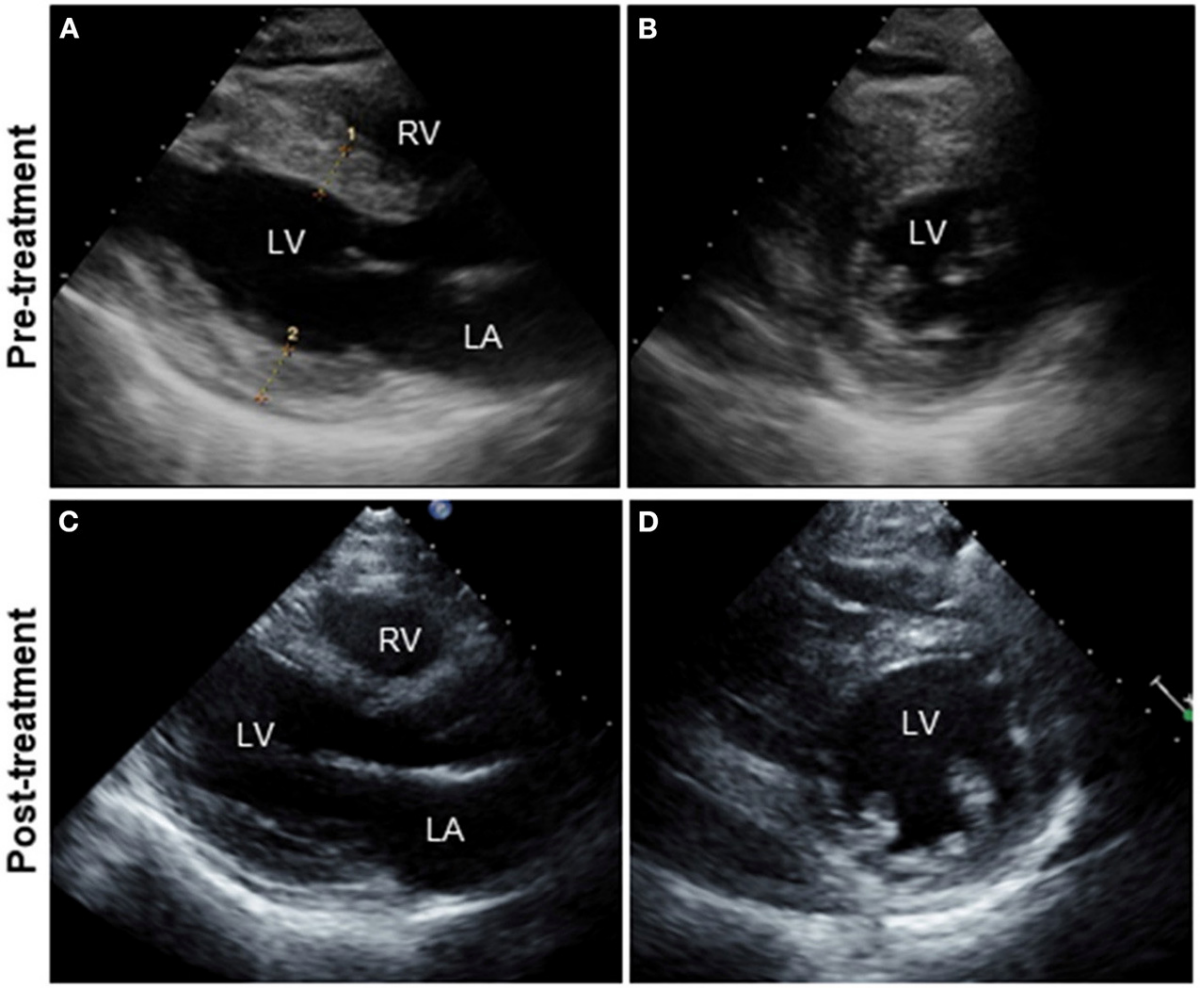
Serial TTE. Parasternal long-axis (A,C) and short-axis views (B,D). TTE on admission showing diffuse and symmetrical LV wall thickening (13mm), decreased cavity size (LVDd: 39 mm) with preserved LVEF (52%), and pericardial effusion (A,B). Follow-up echocardiography on day 4 after intravenous pulse steroid therapy reveals a rapid and dramatic decrease in LV wall thickness (7 mm) and concomitant increases in LVDd (45 mm) and LVEF (72%) (C,D). The improvement in pericardial effusion can be noted. TTE, transthoracic echocardiography; LA, left atrium; LV, left ventricle; RV, right ventricle; LVDd, left ventricular end-diastolic diameter; LVEF, left ventricular ejection fraction.
